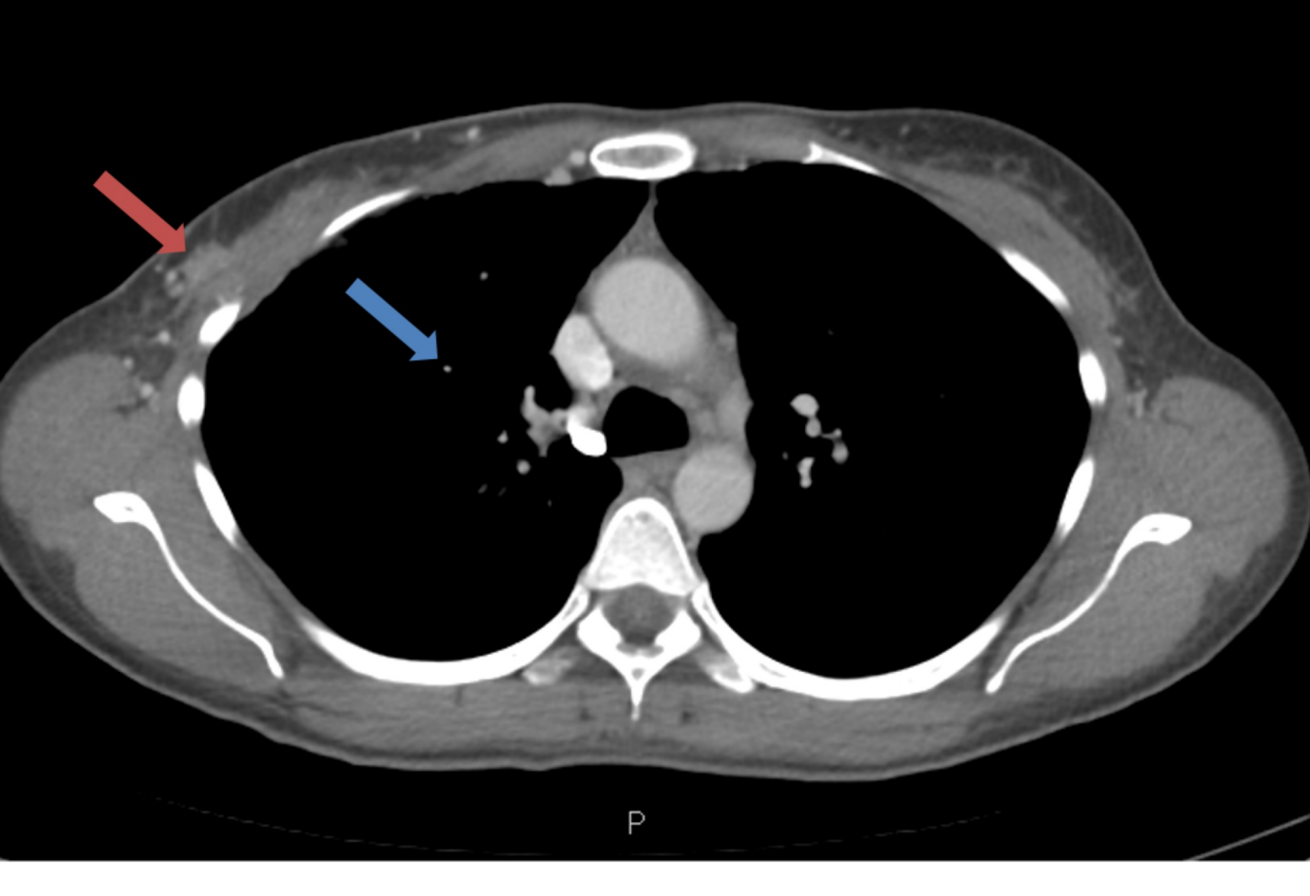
Computed tomography (CT) of the chest with intravenous and oral contrast. CT of the chest showing 1) 8 mm right upper lobe nodule suspicious for metastatic disease and 2) 6 x 12 mm enhancing area in lateral aspect of right breast which may be residual or recurrent disease.
